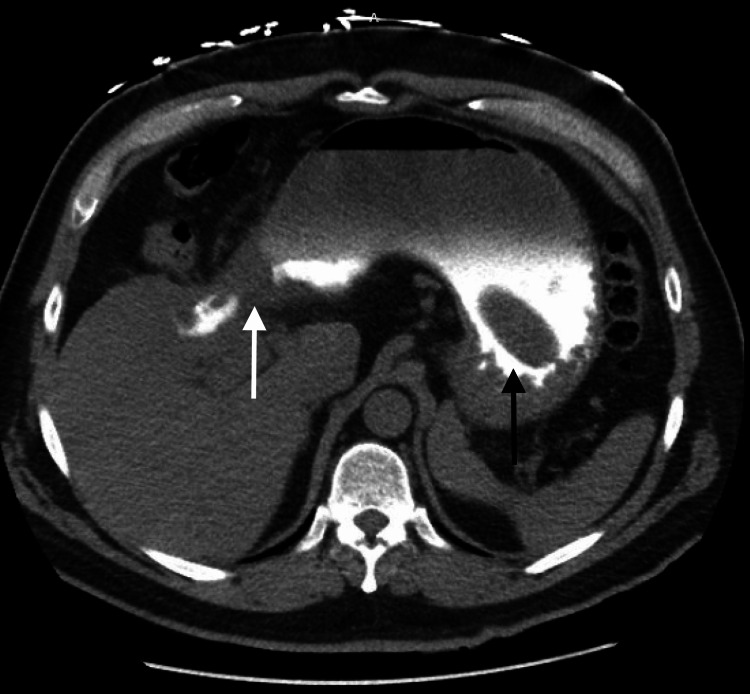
Axial CT of the abdomen showing contrast crossing a cholecystoduodenal fistula (white arrow) and a 6 x 3-cm gallstone in the lumen of the stomach (black arrow)
